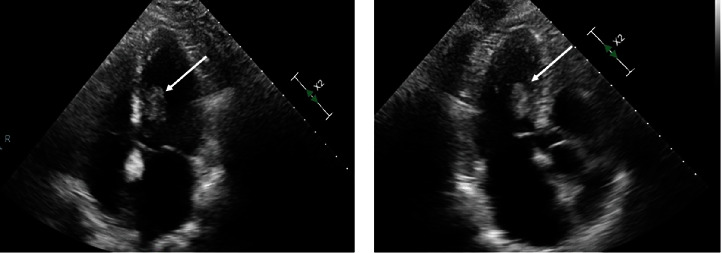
Transthoracic echocardiogram. Panels (a) (apical 4-chamber view) and (b) (apical 3-chamber view) show an echogenic mass located inside the left ventricle attached to the interventricular septum.
